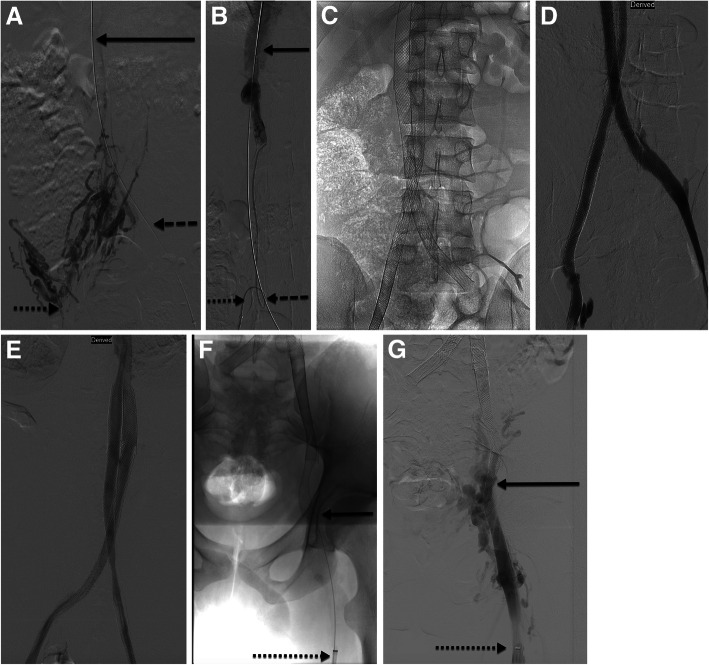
18 y/o M with chronic right lower extremity swelling and DVT presents for planning venogram after MRI demonstrated IVC agenesis from the level of the external iliac to the suprarenal IVC. a Initial venogram from the right groin shows numerous diminutive collateral vessels (dotted arrow). A wire was able to be passed from the left groin through collateral vessels (dashed arrow) to the suprarenal IVC (solid arrow). b Wires and catheters were eventually able to be passed through retroperitoneal collaterals until the bilateral accesses reached the suprarenal IVC (right groin access marked by dotted arrow, left groin access marked by dashed arrow, IVC solid arrow). c IVC reconstruction with stent complex placed from bilateral groins to the suprarenal IVC. d Final venogram through stent complex showed excellent outflow with no intrastent stenosis or delay in transit of contrast. e Follow up venogram at POD 45, demonstrated continued excellent outflow with no intrastent stenosis or occlusion. Patient’s symptoms of right lower extremity swelling was completely resolved. f Follow up venogram 5 months following stent placement, patient had begun to have difficulty with anticoagulation compliance and exhibited symptoms of leg swelling. Unable to pass a wire or catheter beyond the area marked with the solid arrow. g Accompanying venogram depicting complete occlusion of the right stent complex with chronic thrombus and numerous collateral vessels draining the right lower extremity (Note, patient is positioned prone). On IVUS, there was kinking of the stent complex near the femoral head which likely was at least partially contributing to the stent complex failure
